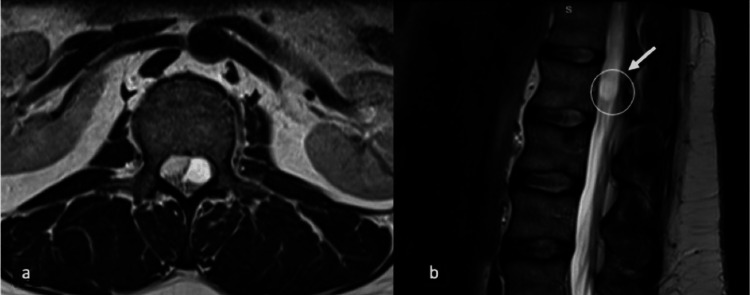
(A) Axial and (B) sagittal T2 weighted MRIs of the lumbar spine show a round extramedullary, intraspinal cystic lesion at the junction between the conus medullaris and cauda equina at L1 level causing mild displacement of the conus medullaris and cauda equina contralateral side. MRI, magnetic resonance image
